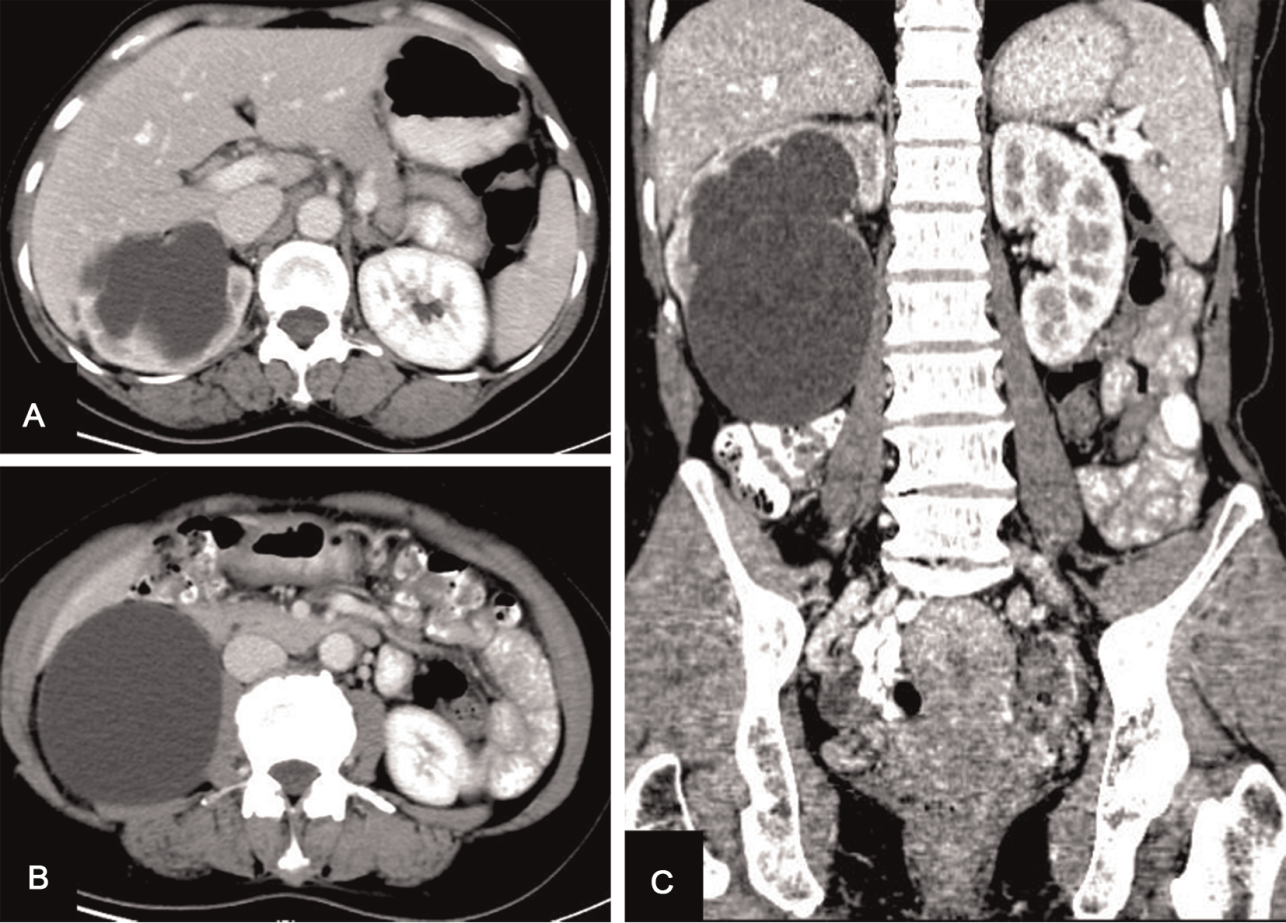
Enhanced CT scan showing a lower pole cyst of the right kidney of approximately 78 mm × 86 mm size and hydronephrosis of the right kidney with reduced renal parenchymal perfusion. (A,B) Sagittal images, (C) coronal image. CT, computed tomography.
